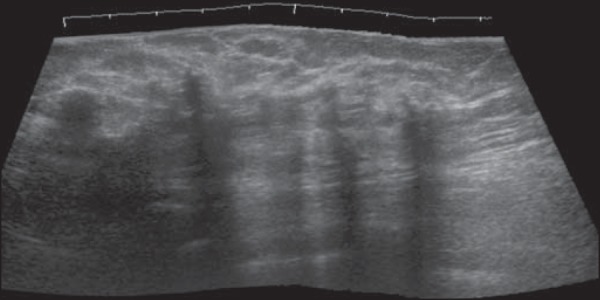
Ultrasound of the left breast, showing an area of architectural distortion
